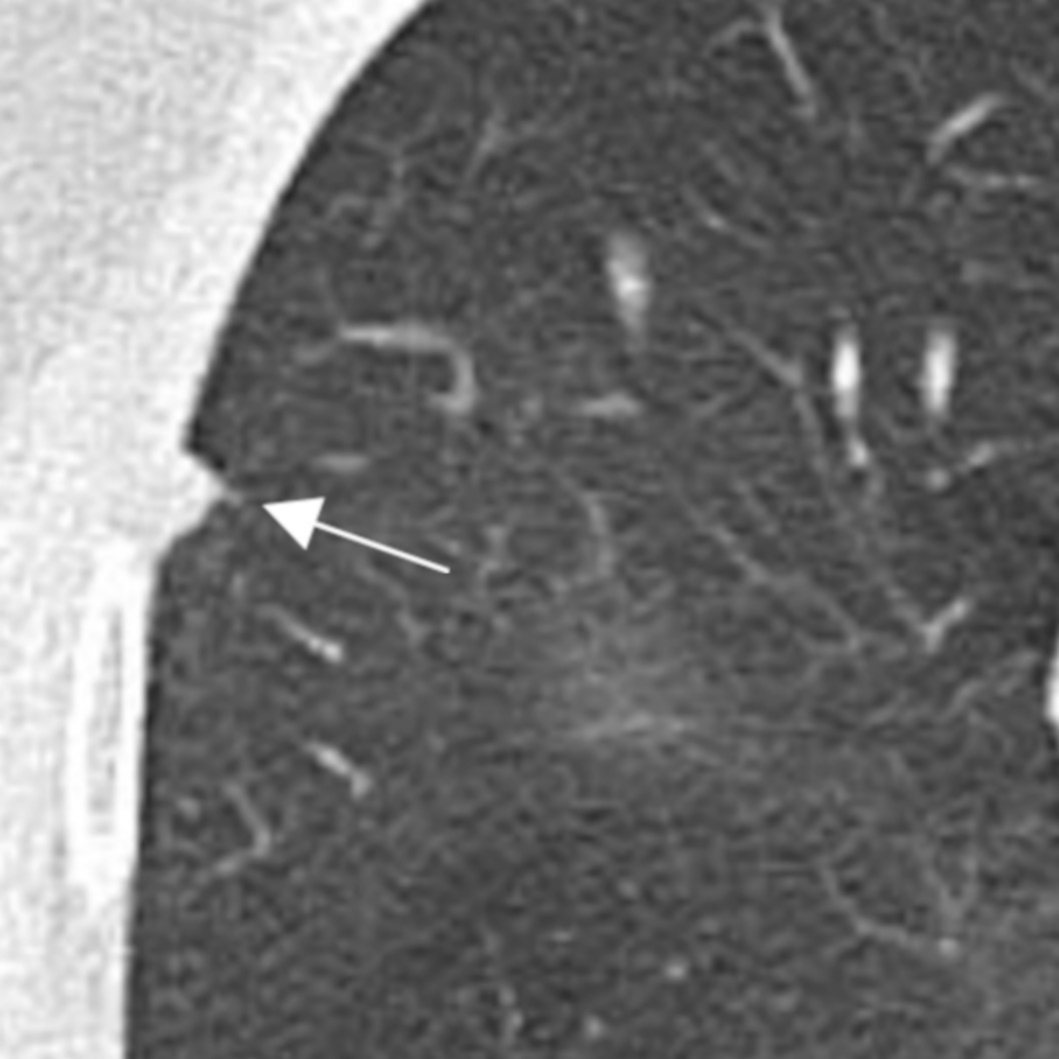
Axial non‐contrast CT. Eight millimetre juxtapleural nodule in the right middle lobe (white arrow). Triangular morphology, position relative to the pleura and size less than 10 mm indicate benignity. This reflects a normal intrapulmonary lymph node.
